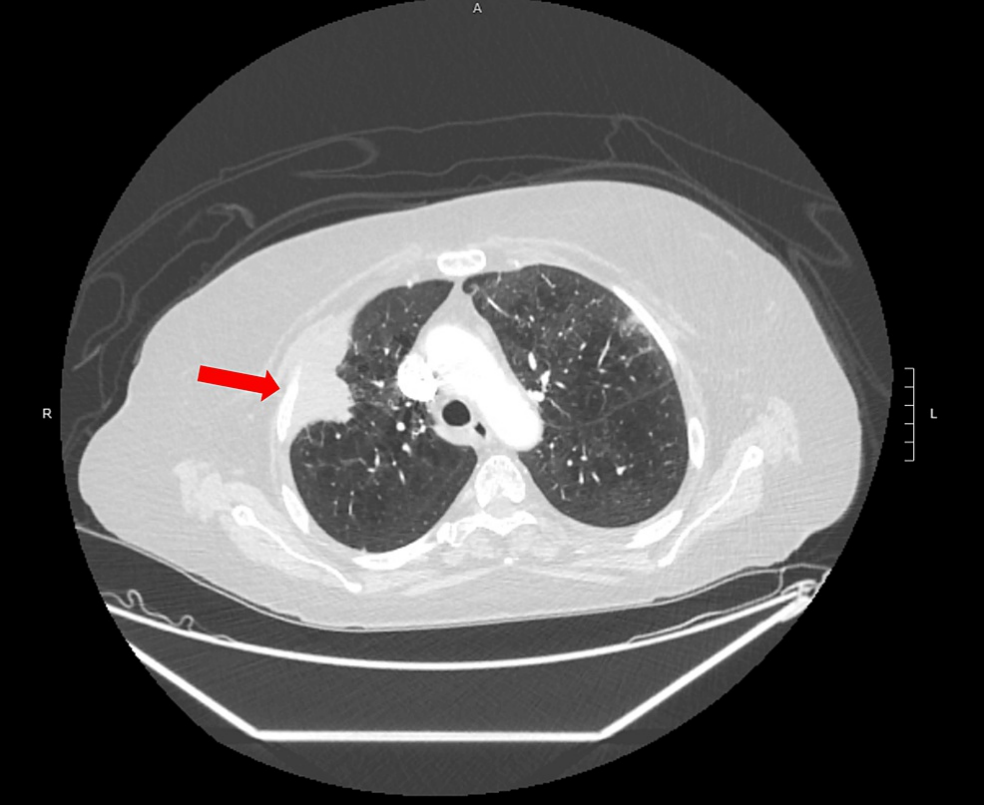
Chest CT scan showing a 6.8 x 3.5 cm mass in the right upper lobe of the lung
